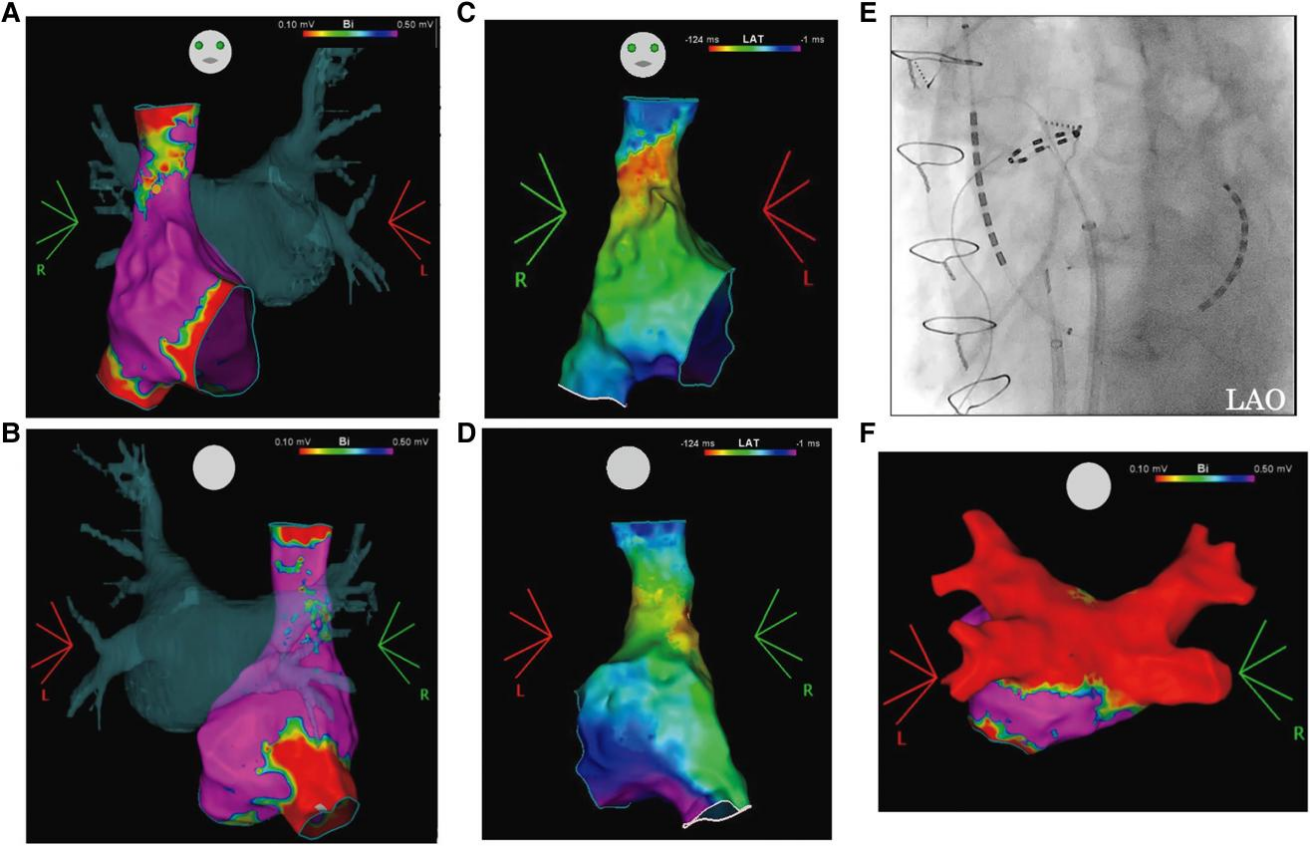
(*A* and *B*) Pre-ablation right atrial voltage map merged with left atrial computed tomography image. A myocardial sleeve extending from the superior vena cava was observed, reaching up to 35.6 mm from the superior border of the sinus node (yellow tag). (*C* and *D*) Pre-ablation right atrial activation map merged with left atrial computed tomography image. (*E*) Fluoroscopic images of pulsed field ablation (PFA) of the right superior pulmonary vein using a PulseSelect catheter. (*F*) Post-pulsed field ablation left atrial voltage map showing low voltage in the pulmonary veins and posterior wall.
